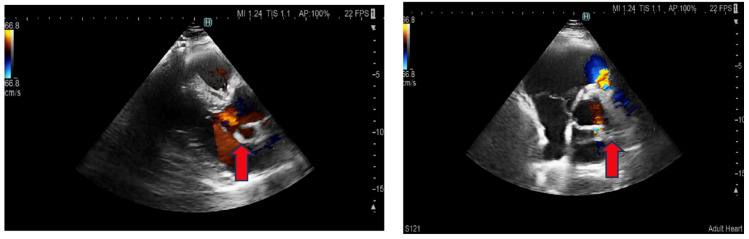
Transthoracic echocardiography: Parasternal long and short axis. The red arrow shows a pseudoaneurysm.
